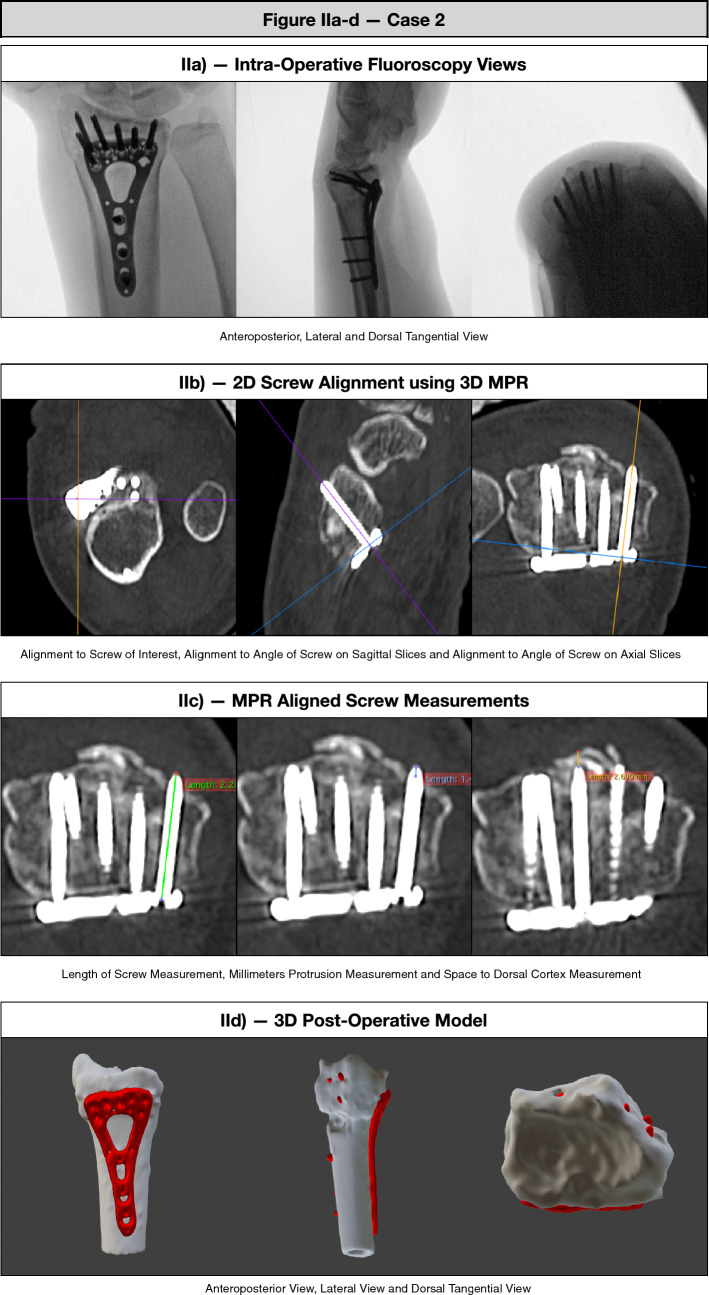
a Intra-operative anteroposterior, lateral and dorsal tangential fluoroscopy views; b alignment to screw of interest, angle of screw on sagittal slices and angle of screw on axial slices; c length of screw measurement, millimetres protrusion measurement and space to dorsal cortex measurement; d 3D model of post-operative CT scan with volar, radial and articular views
